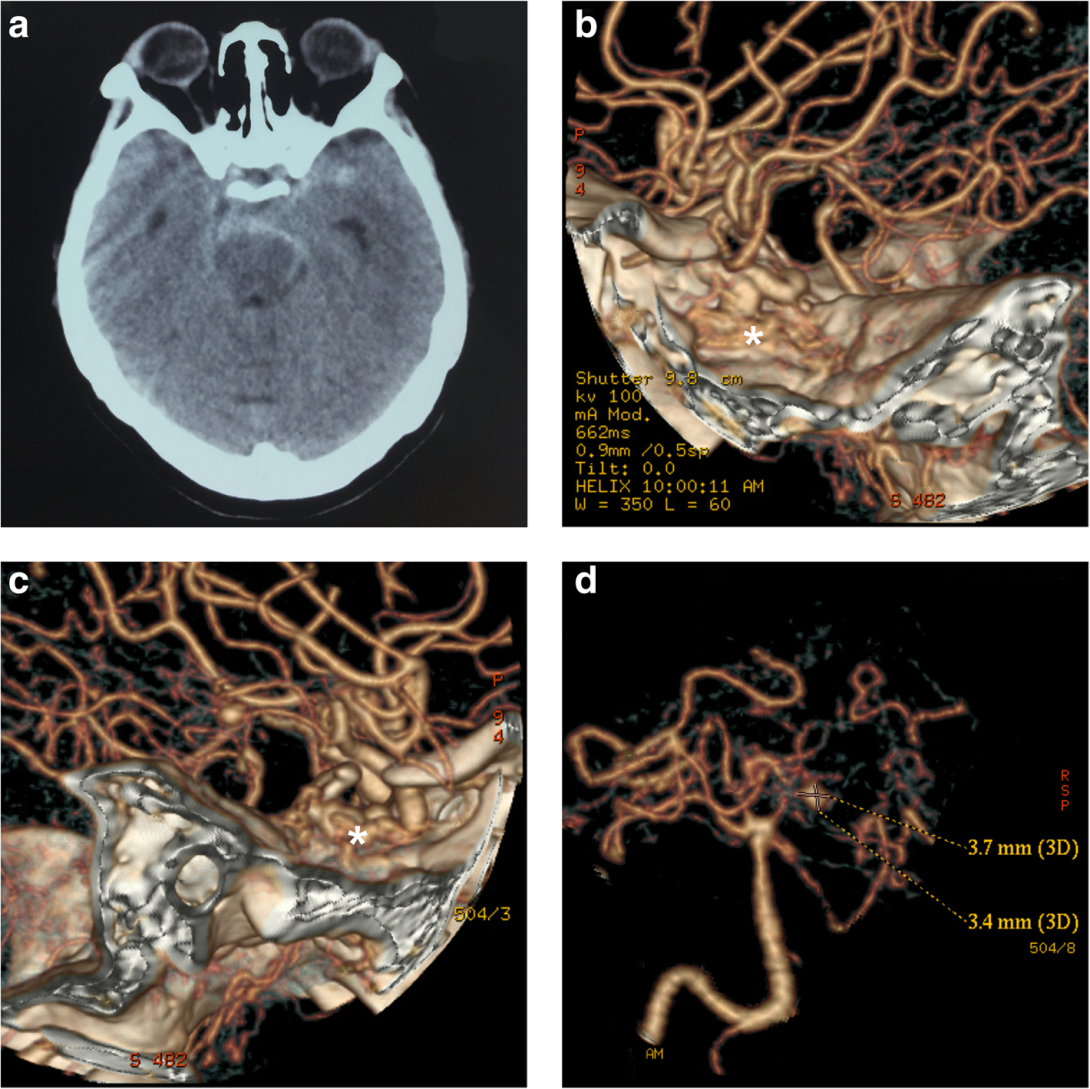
a: A head CT scan shows an SAH concentrated in the perimesencephalic cistern. b-c: CTA shows that the cavernous portion of the bilateral internal carotid arteries (ICAs) is absent (asterisks). The bilateral middle cerebral arteries and anterior arteries are normal. d: An aneurysm is identified in the PCA region. Abbreviations: CT: computed tomography, SAH: subarachnoid hemorrhage, CTA: CT angiography, ICA: internal carotid artery, PCA: posterior cerebral artery
